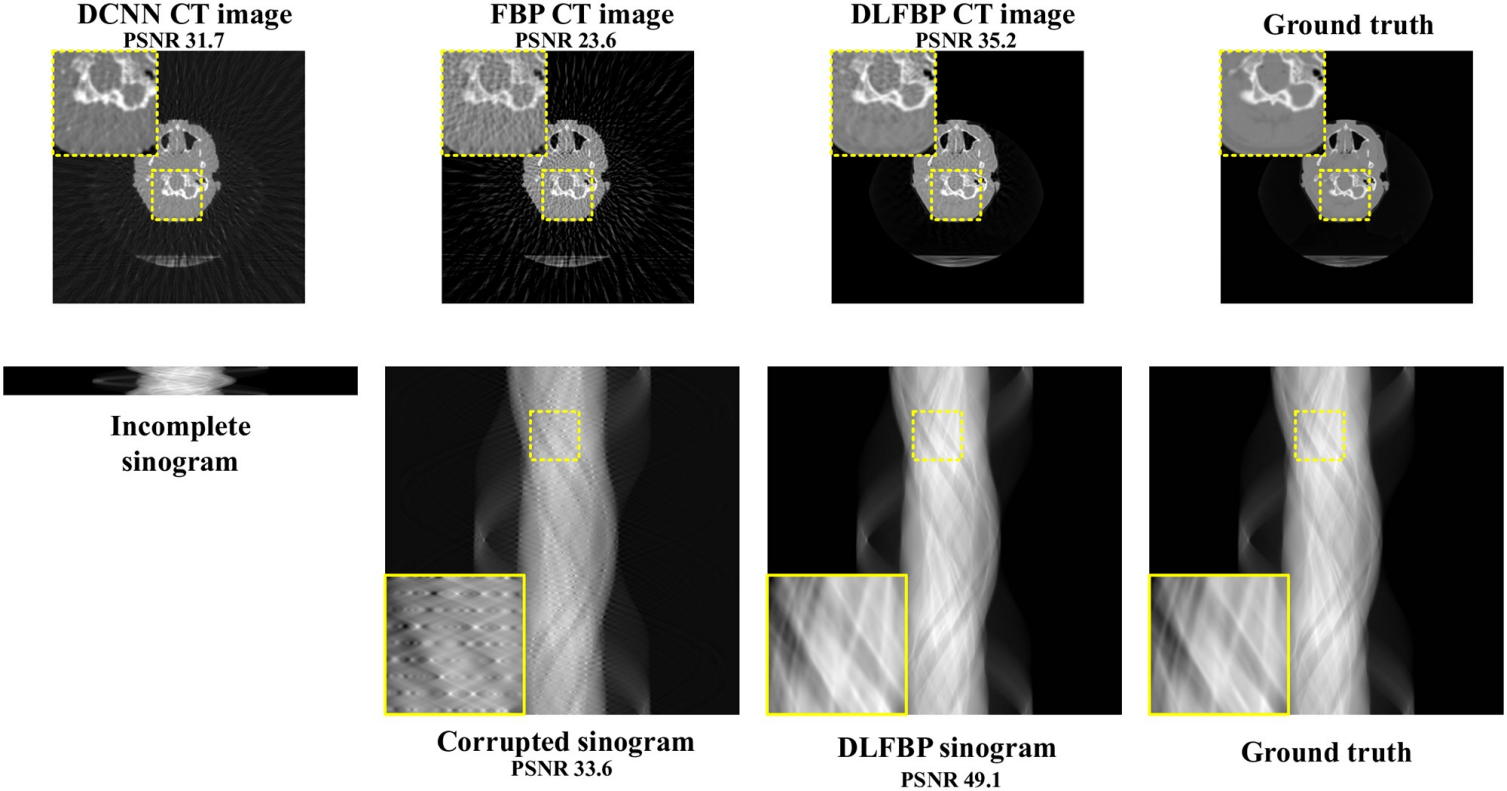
The sparse-view results of one head slice with an incomplete sinogram with 60 views by using DCNN [32], FBP and DLFBP. The incomplete singoram has a size of 60 × 731 pixels. The corrupted sinograms generated by the forward projection have a size of 720 × 731 pixels. All CT images have a size of 512 × 512 pixels after cutting off the surrounding blank region. Some regions of these images, indicated by the yellow box, are enlarged for better visualization.
